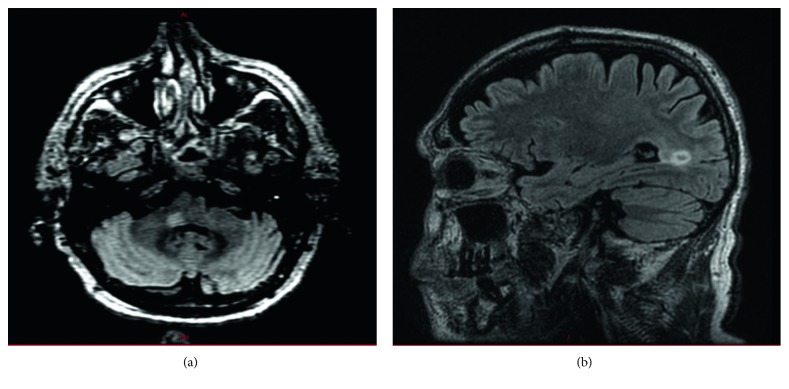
MRI of the brain showing hyperintense lesion in the right middle cerebellar peduncle lesion (a) and ring-shaped periatrial white matter lesion (b).
